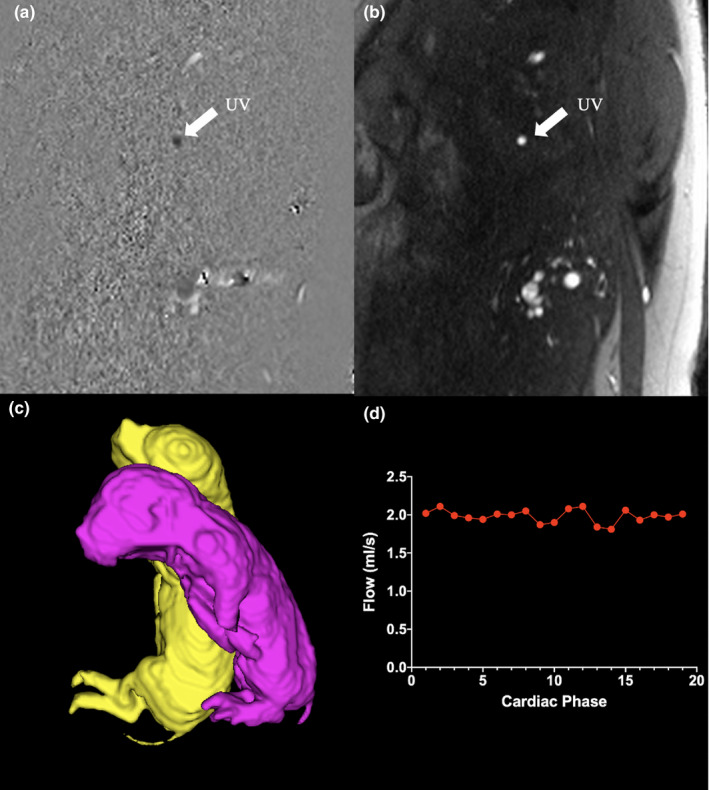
Measurement of umbilical blood flow in utero by cine phase‐contrast MRI. Phase (a) and modulus (b) images from the cine phase‐contrast acquisition, segmentation of fetal volumes for the quantification of fetal weight (c), and typical non‐pulsatile flow profile obtained from the umbilical vein (d)
